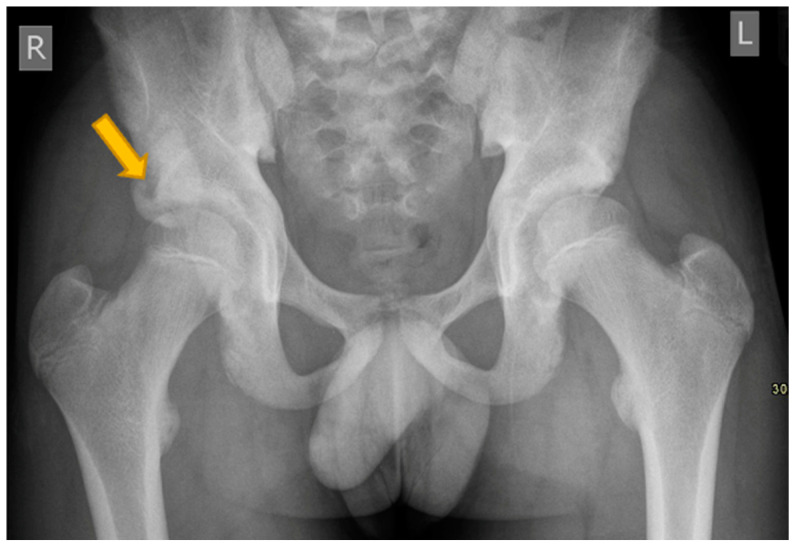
Painful right-sided limp in a 14-year-old male, a keen footballer. A plain radiograph shows an acute avulsion fracture of the right anterior inferior iliac spine.
